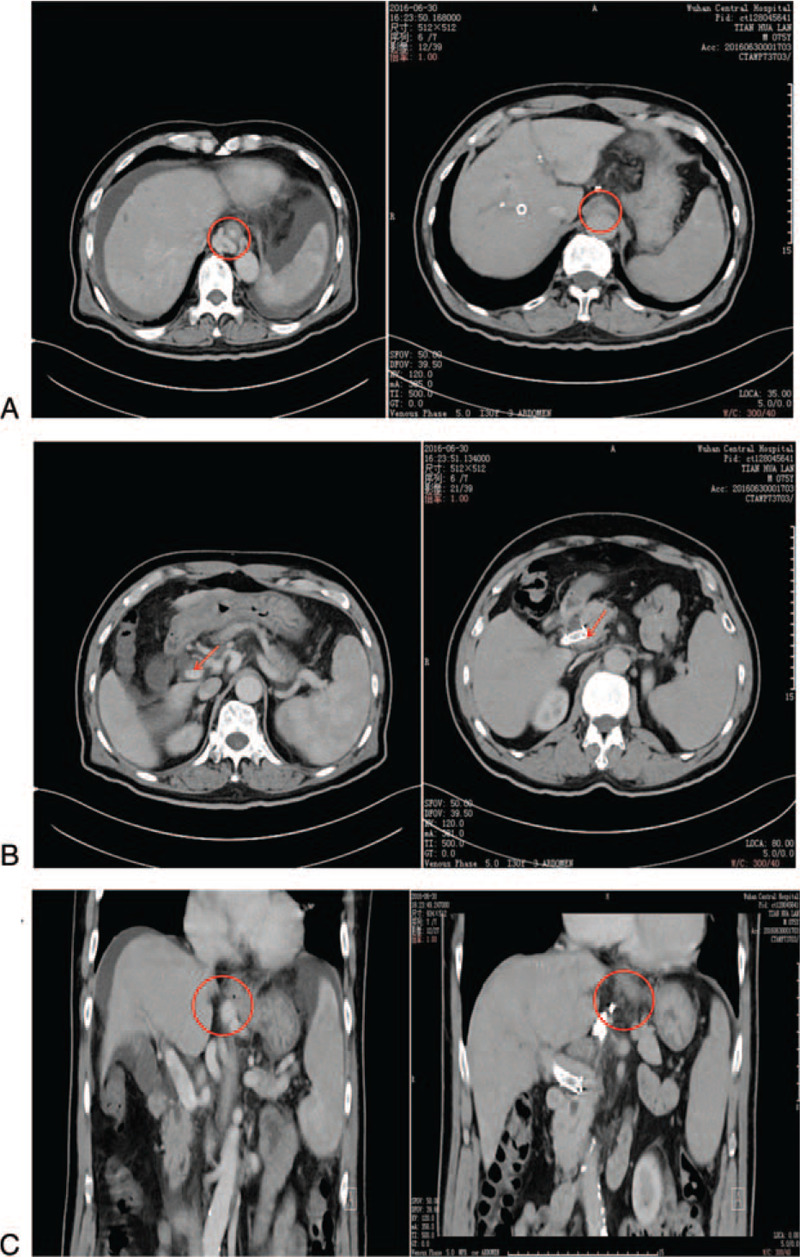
CT scans before and after TIPS treatment. (A). Esophageal varices before (left) and after (right) TIPS treatment. The red circle indicates esophageal varices. (B). Portal vein thrombosis before (left) and after (right) TIPS treatment. The red arrow indicates the portal vein. C. Gastric coronary vein varices before and after TIPS treatment. The red circle indicates the gastric coronary vein. CT = computed tomography.
